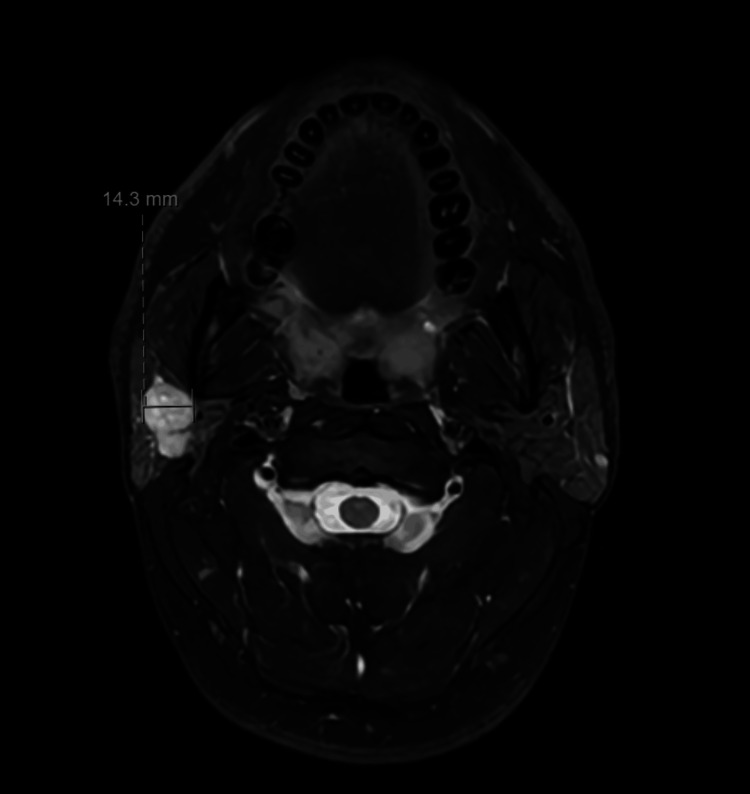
Axial MRI neck showing parotid lesion
